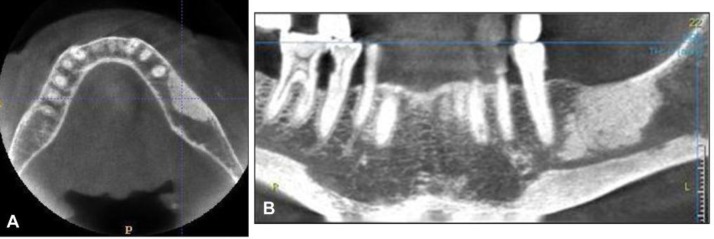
A = Axial images of the mandible obtained with CBCT unit showing thickening of ‎cortical plate and focal region of medullary bone density on the left body of mandible, the ‎overlying mucosa is clinically intact‏.‏ B = Two-dimensional multiplanar reconstruction image showing the vertical dimension of the ‎region of osteosclerosis and the relationship with mandibular canal.
